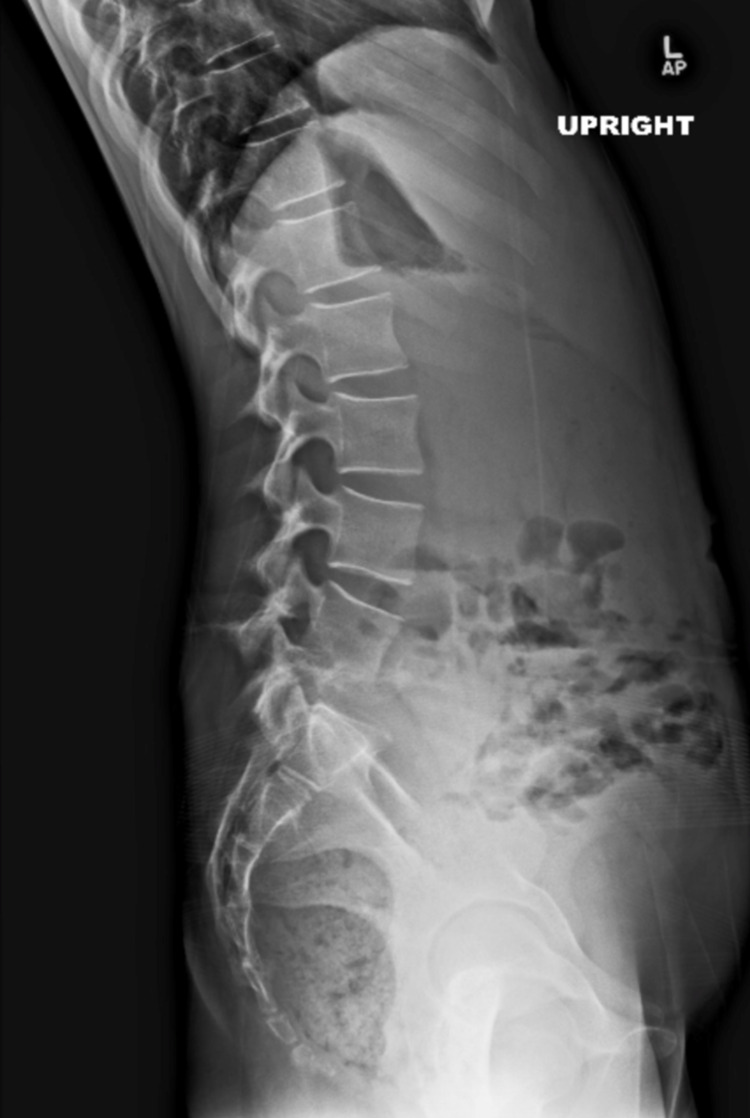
A lateral lumbar spine x-ray of a 12-year-old female The image shows a lateral lumbar spine x-ray of a 12-year-old female that presented to our institution with a four-month history of back pain, showing loss of the disc space and junctional kyphosis at L4/L5. She was diagnosed with tuberculosis of the lumbar spine.
